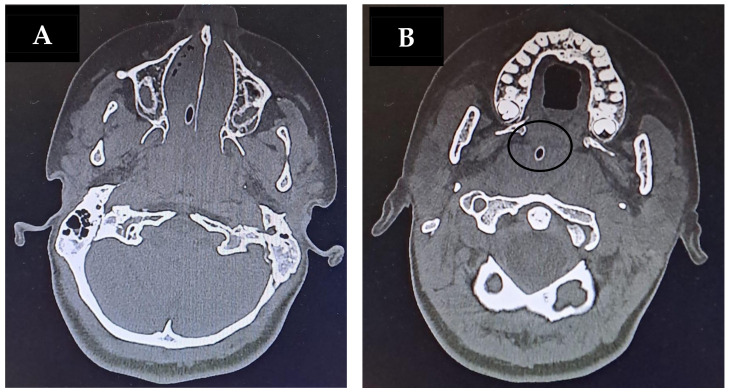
Case 1. Axial CT scan showing obstruction of the pharyngeal lumen at the upper (A) and lower (B) third of the oropharynx due to tonsillar hypertrophy. The circle highlights the nasogastric tube, compressed by hypertrophic tonsillar tissue.
